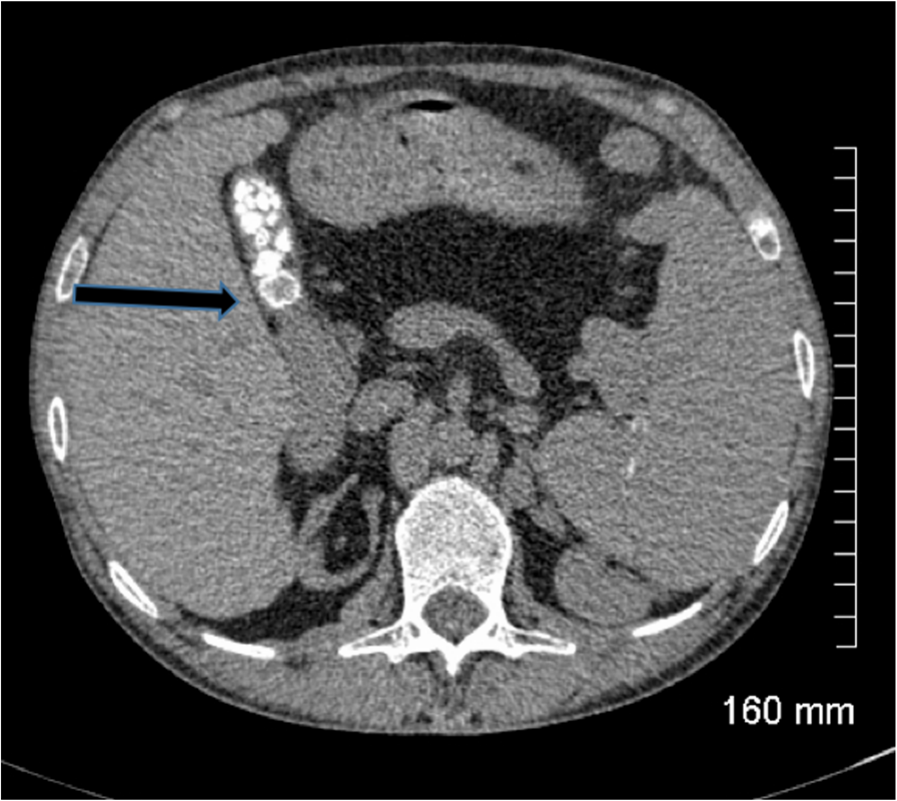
Axial contrast-enhanced CT of the abdomen. There are several calcified gallstones (arrow) within the gallbladder which are hyper attenuating relative to surrounding bile
